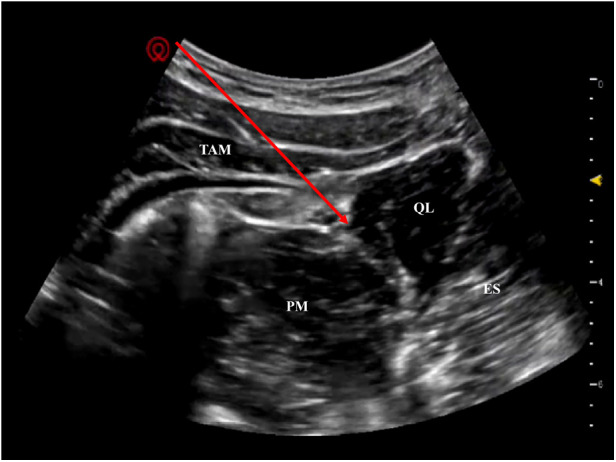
Ultrasound imaging of the target fascial space for type Ⅱ QLB in a 19-month-old infant. QL, quadratus lumborum; ES, erector spinae; TAM, transversus abdominis muscle; PM, psoas major muscle (red arrow indicates the target fascial space for type Ⅱ QLB).
